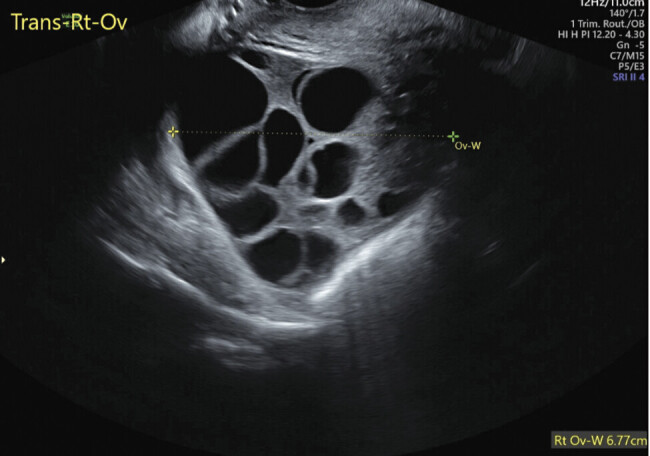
Transvaginal ultrasound of theca lutein cysts within ovary at the time of partial hydatidiform mole diagnosis.
